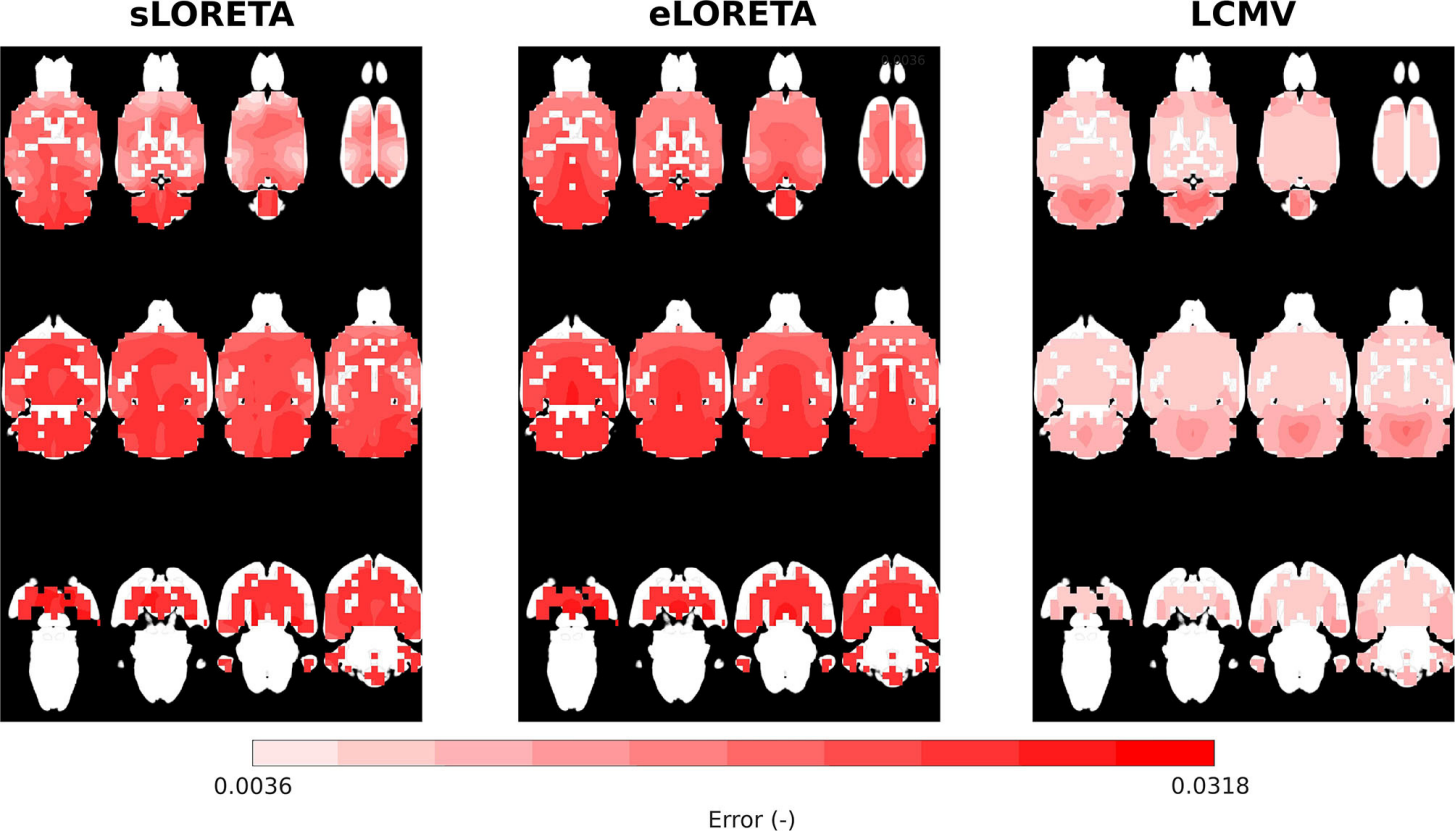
Reliability maps interpolated on MRI scan axial slices for eLORETA, LCMV, and sLORETA inverse solvers with SNR = 25 dB.
